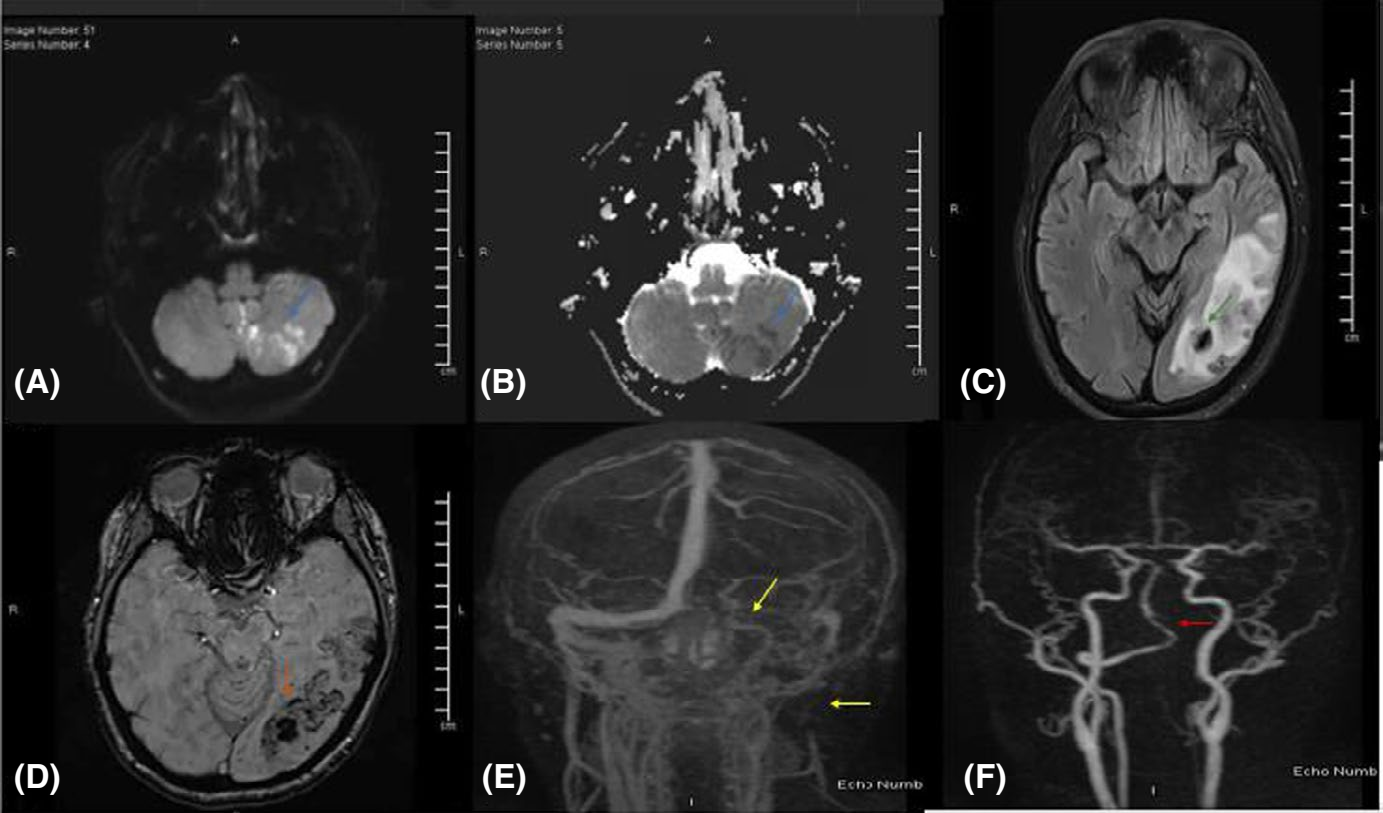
(A‐F) MRI/MRV/MRA head. (A, B) There is diffusion restricting (Blue arrow) and slightly FLAIR hyperintense acute infarcts in the left postero‐inferior cerebellar hemisphere (Green arrow). (C, D) SWI shows blooming in the left temporo‐occipital suggestive of venous hypertension with hemorrhage, with surrounding edema evident on FLAIR hyperintense (Orange arrow). (E, F) MRA, show occlusion of intracranial V4 and skull base V3 segments of the left vertebral artery (Red arrow). MR venogram shows that there is interval near total recanalization of the left sigmoid sinus, partial recanalization of the left internal jugular vein and significant amount of residual clot in the left transverse sinus medially with recanalization of only its lateral aspect (Yellow arrows)
